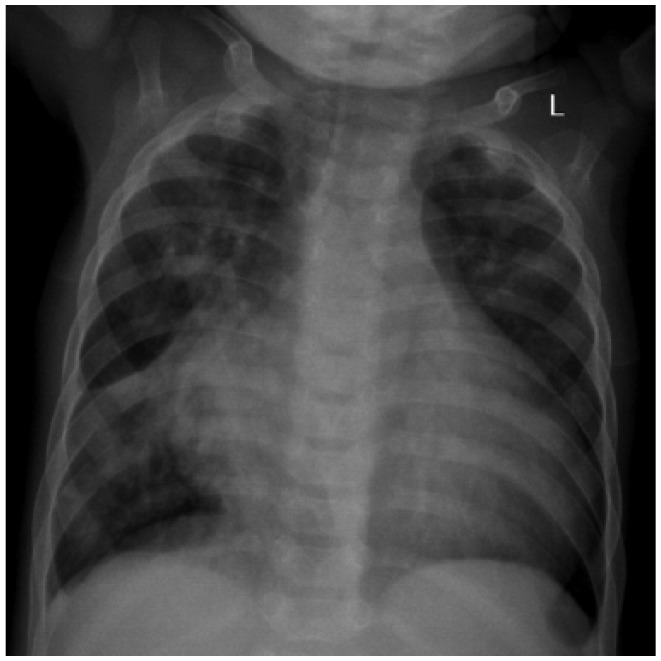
Chest X-ray (AP view) displaying cardiomegaly, increased vascular markings, and right lower lobe consolidation.
